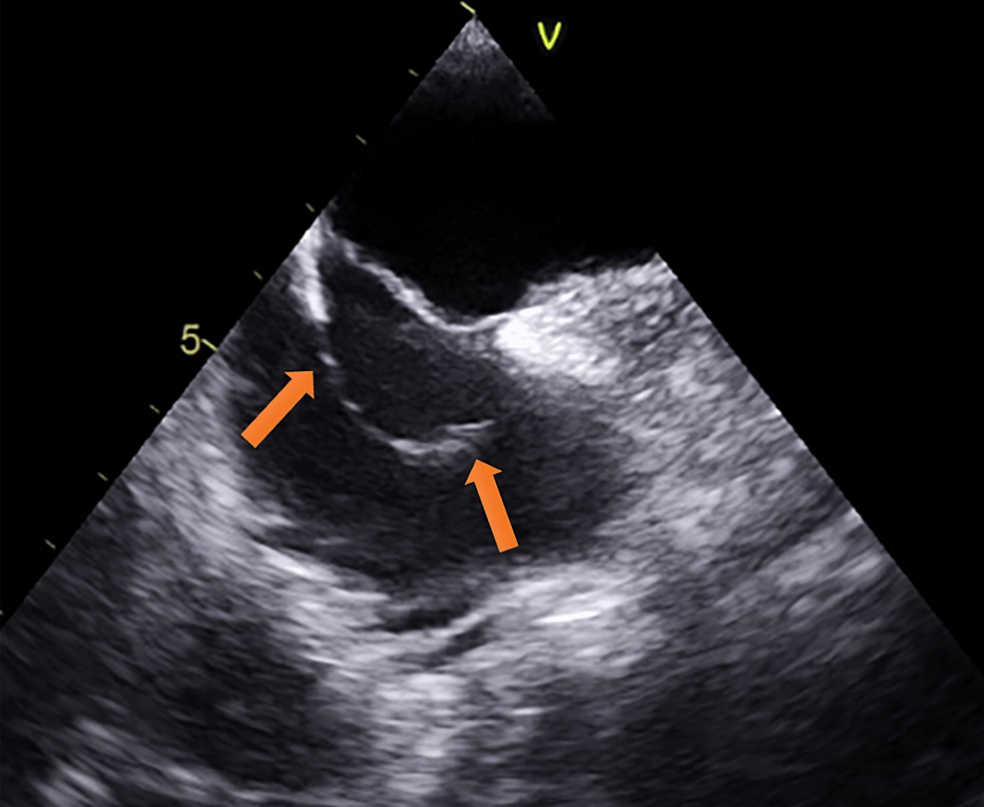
A TEE demonstrating a persistent eustachian valve extending from the inferior vena cava to the superior vena cava (arrows). TEE: Transesophageal Echocardiogram
